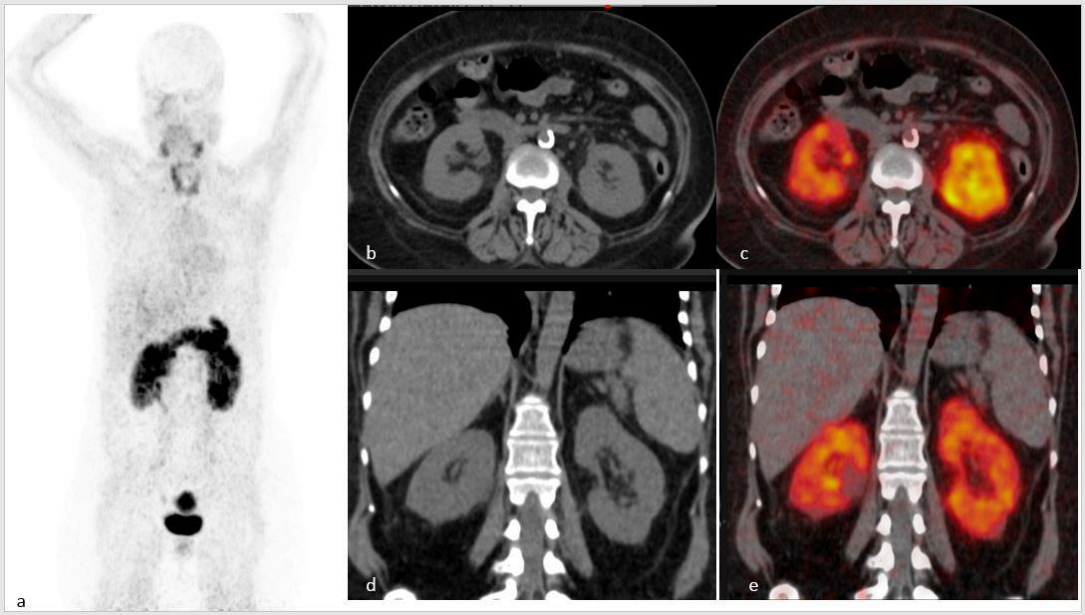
MIP image of ^68^Ga-FAPI PET CT shows increased radiotracer uptake in both kidneys. Physiological tracer uptake in the pancreas and myometrium. Increased tracer uptake in the thyroid is likely to be related to thyroiditis (a). Trans-axial CT and fused PET CT images (b and c, respectively), as well as coronal CT and fusion PET CT images. (d and e) show avid inhomogeneous tracer uptake in both kidneys suggestive of inflammatory etiology
